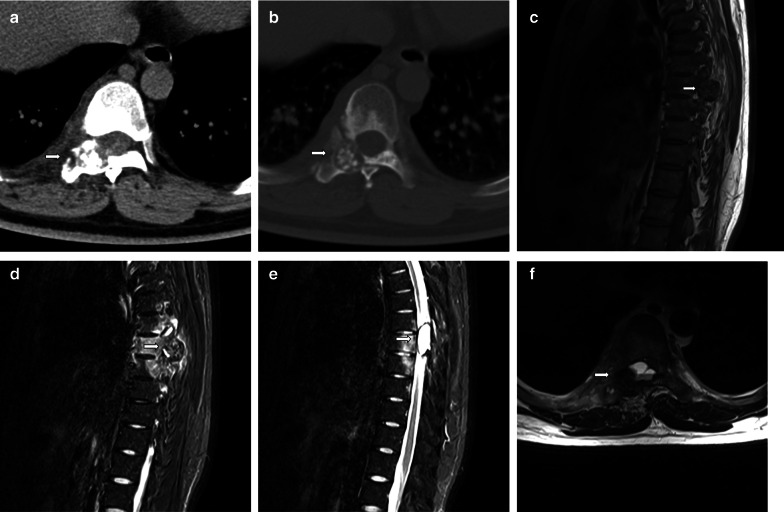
A 28-year-old man with aneurysmal bone cyst (ABC). A 28-year-old man with a lesion on the right accessory. The nidus can be visualized well on axial CT (a, b), especially in the bone window (b). The nidus exhibits isointense signal on T1WI (c) and hyperintense signal on FS T2WI (d). ABC appears conspicuous on MRI (e, f), with a typical finding of the fluid–fluid level (f)
